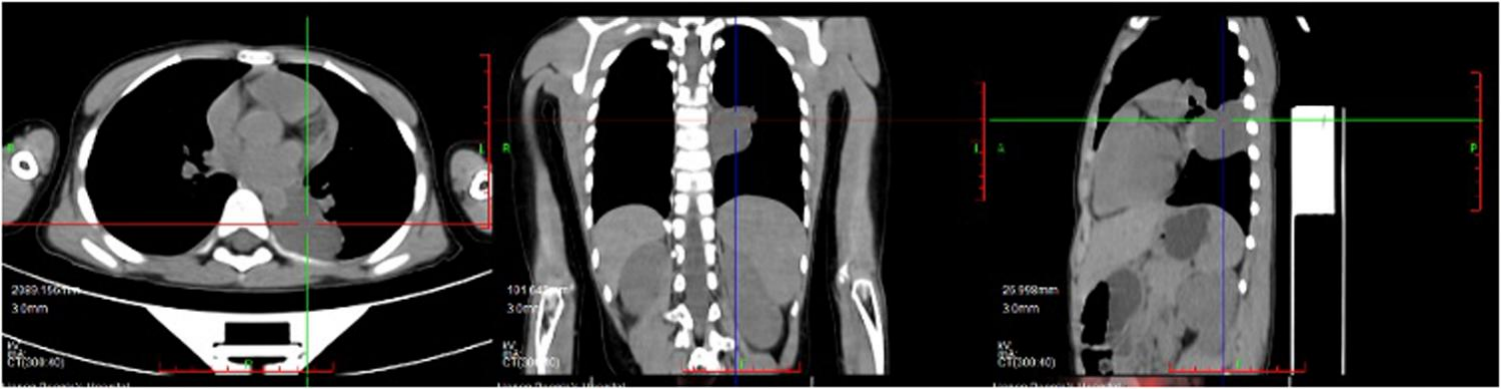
Preoperative PET/CT indicated paraspinal cystic lesion in the left lower lobe with loss of metabolism.
